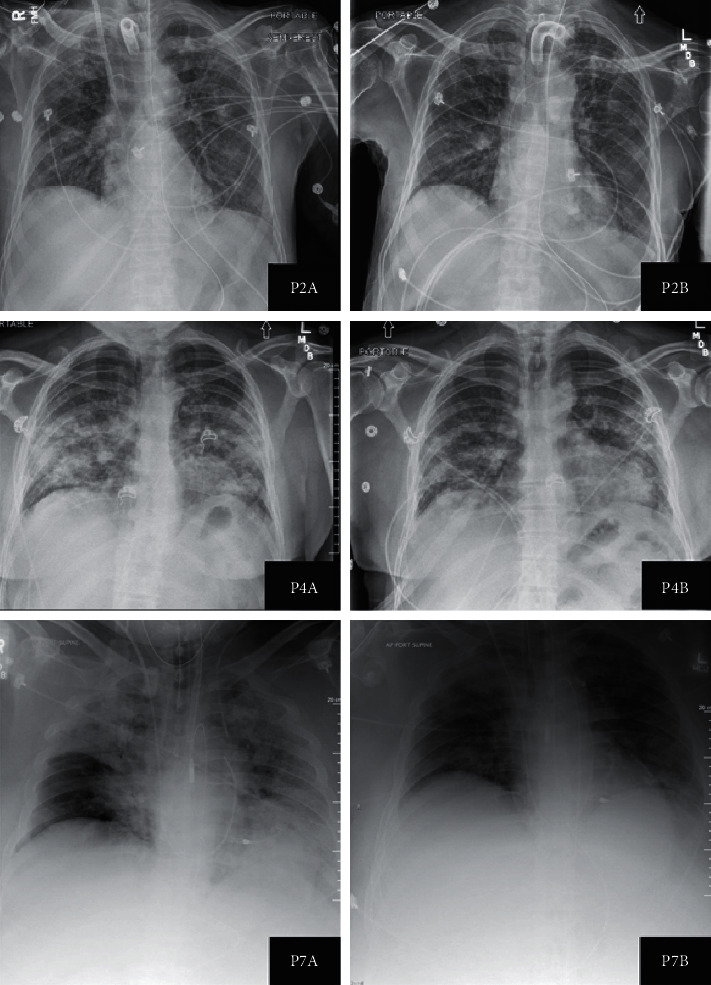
Chest X-rays of patients II, IV, and VII before (A) and after (B) treatment with dornase alfa. Imaging demonstrates improved resolution of opacities including for those who had prolonged mechanical ventilation (patients II, P2A/B, and VII, P7A/B) and for those not requiring mechanical ventilation (patient IV, P4A/B).
